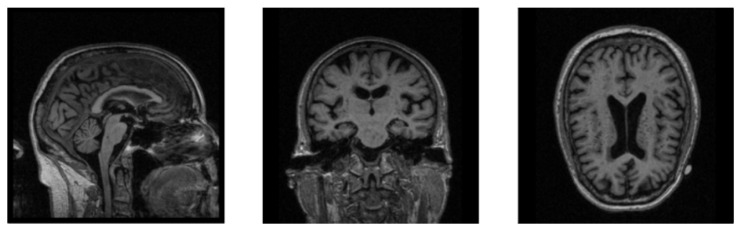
The sectional views of a 3D MRI image along three directions. The three views from left to right are sagittal, coronal, and axial.
